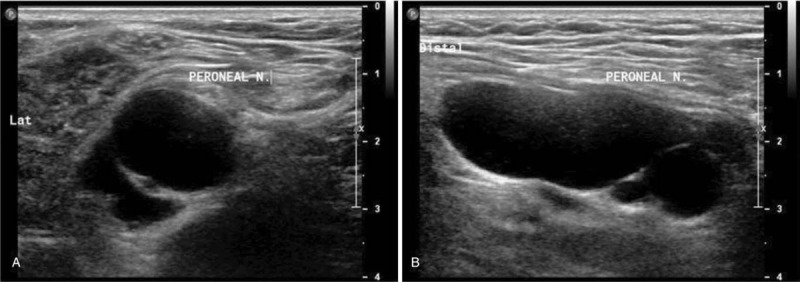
Transverse (A) and longitudinal (B) views of ultrasonography show an approximately 19 × 16 × 40 mm sized hypoechoic cystic mass located at the upper lateral component of the left popliteal fossa.
